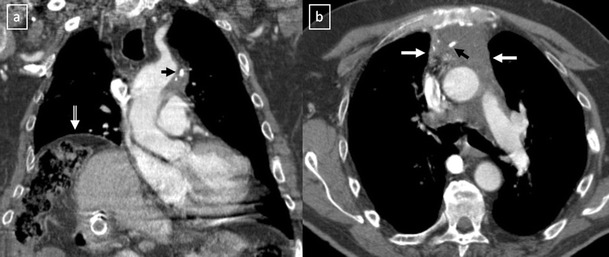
Idiopathic fibrosing mediastinitis in a 64-year-old man. a Coronal multiplanar reconstruction and axial b constrast-enhanced CT scan show an infiltrating soft tissue mass (*white arrows*) in mediastinum encasing major vessels. Punctate calcifications are observed (*black arrows*) as well as an elevation of the right hemidiaphragm (*open arrow*) secondary to the phrenic nerve involvement
